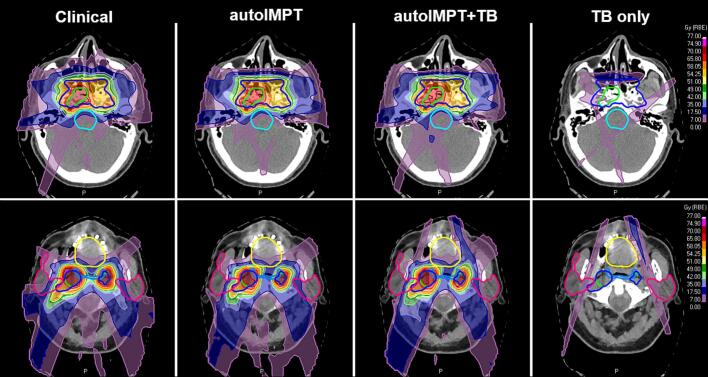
Transversal CT slices with typical dose distributions of the clinical, autoIMPT, autoIMPT+TB, and the TB-only contribution (11% of the total spots for this patient) to the autoIMPT+TB plan, for one patient (patient 11). Delineations: green=CTV7000, dark blue=CTV5425, light blue=brainstem, yellow=oral cavity, pink=parotids. In the automated plans, the brainstem and parotids receive less dose. (For interpretation of the references to colour in this figure legend, the reader is referred to the web version of this article.)
